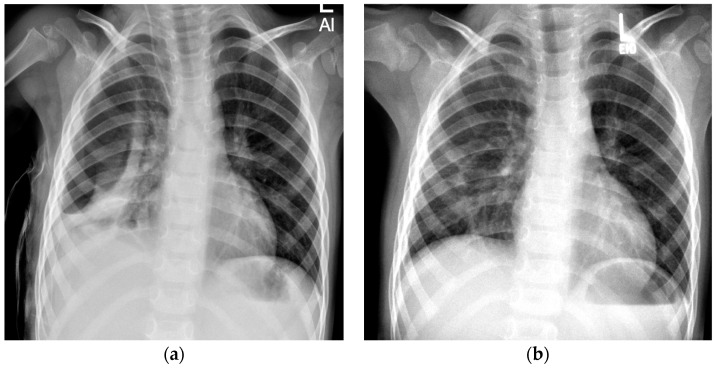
Spontaneous resorption of a pneumothorax in a 3-year-old boy. (a) Anterior–posterior (AP) chest radiograph taken at the time of discharge reveals a right-sided pneumothorax. (b) Spontaneous resorption of the pneumothorax at 2-month follow-up.
